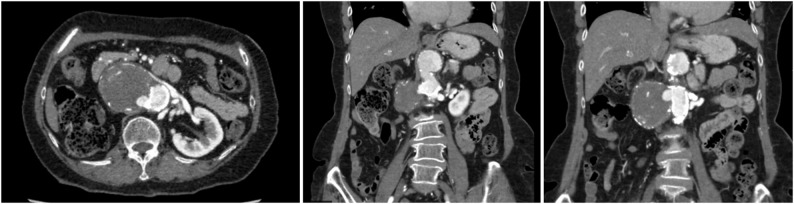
The computed tomography angiography showed the right renal artery aneurysm. Blood inflow is detected inside it from the abdominal aorta. The right kidney is severely atrophic
